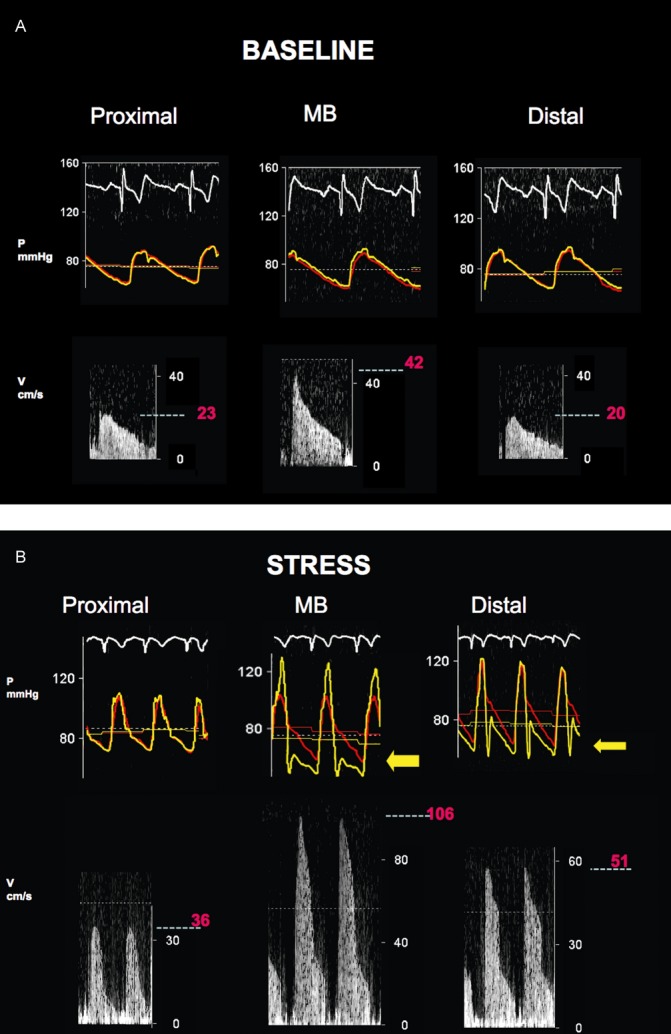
Representative readings of flow velocity and pressure. At baseline (A) Doppler flow velocity and pressure data are shown for one representative patient proximal, within, and distal to the myocardial bridge (MB). Pressure in the aorta (red pressure line) vs the coronary artery (yellow pressure line). Doppler velocity profiles show maximum peak velocity readings at rest of 23, 42 (with prominent “fingertip”), and 20 cm/s, respectively. With peak stress (B), there is no significant change in the 2 pressure lines proximally. Intrabridge (MB) there is systolic overshoot (yellow line) and diastolic decline in pressure, resulting in a diastolic fractional flow reserve (dFFR) of 0.74 (large yellow arrow). Distal to the bridge, there is diastolic pressure recovery with a dFFR of 0.88 (small yellow arrow). Stress Doppler peak velocities are 36, 106, and 51 cm/s, respectively.
